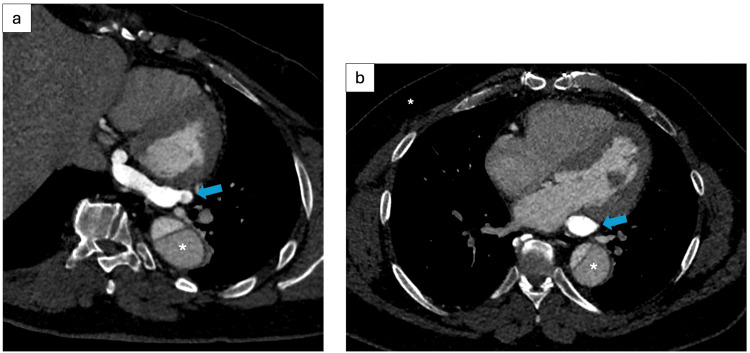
Computed Tomography Imaging of Dilated Coronary Sinus With Early Opacification (a) Axial contrast-enhanced reconstruction along the coronary sinus and (b) axial computed tomography image of the chest demonstrate early contrast opacification of a dilated coronary sinus (arrow), without opacification of the right atrium or contrast mixing, suggesting ostial stenosis. Additionally, a dissection flap is noted in the descending aorta (*).
